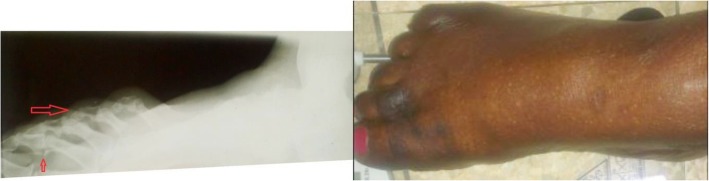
The lateral view foot radiograph and the foot picture of the same patient with CA. The anatomical classification according to Sanders and Frykberg for this foot is type 1 (lesions in forefoot) and 3 (lesions in the tarsal bones). The modified Eichenholtz radiological classification for this foot is stage 1 (foot swelling, fragmentation of subchondral bones in the tarsus, periarticular fracture at the proximal interphalangeal joint of the 2nd toe, toe clawing)
